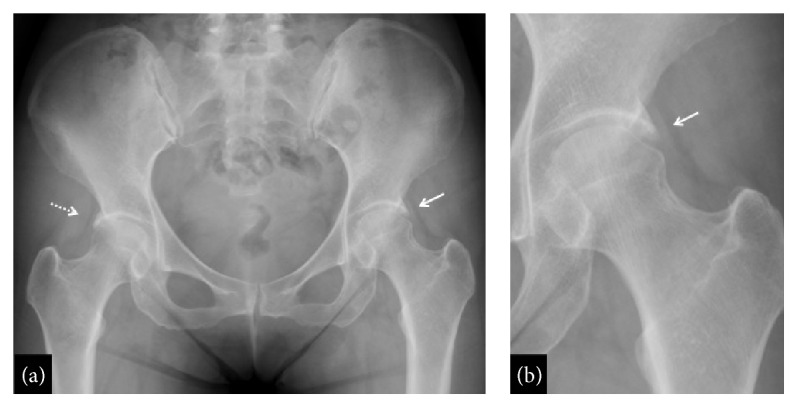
Plain radiographs at the last follow-up. (a) Anteroposterior X-ray obtained at the 2-year follow-up and (b) its enlarged image. Progression of the subchondral collapse was not observed but the acetabular overcoverage in both hips still remained (a dotted arrow indicates right and a white arrow indicates left side of the acetabular overcoverage).
